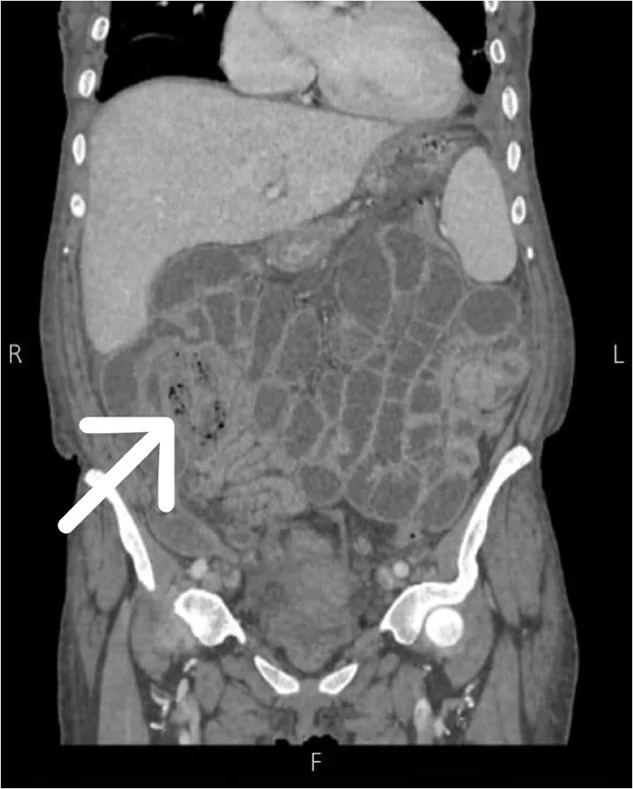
Detailed CT view focusing on the transition point; the arrow indicates the site of obstruction, clearly showing significant proximal bowel dilation (pre-stenotic) and distal bowel collapse, which are hallmark signs of a complete mechanical obstruction.
